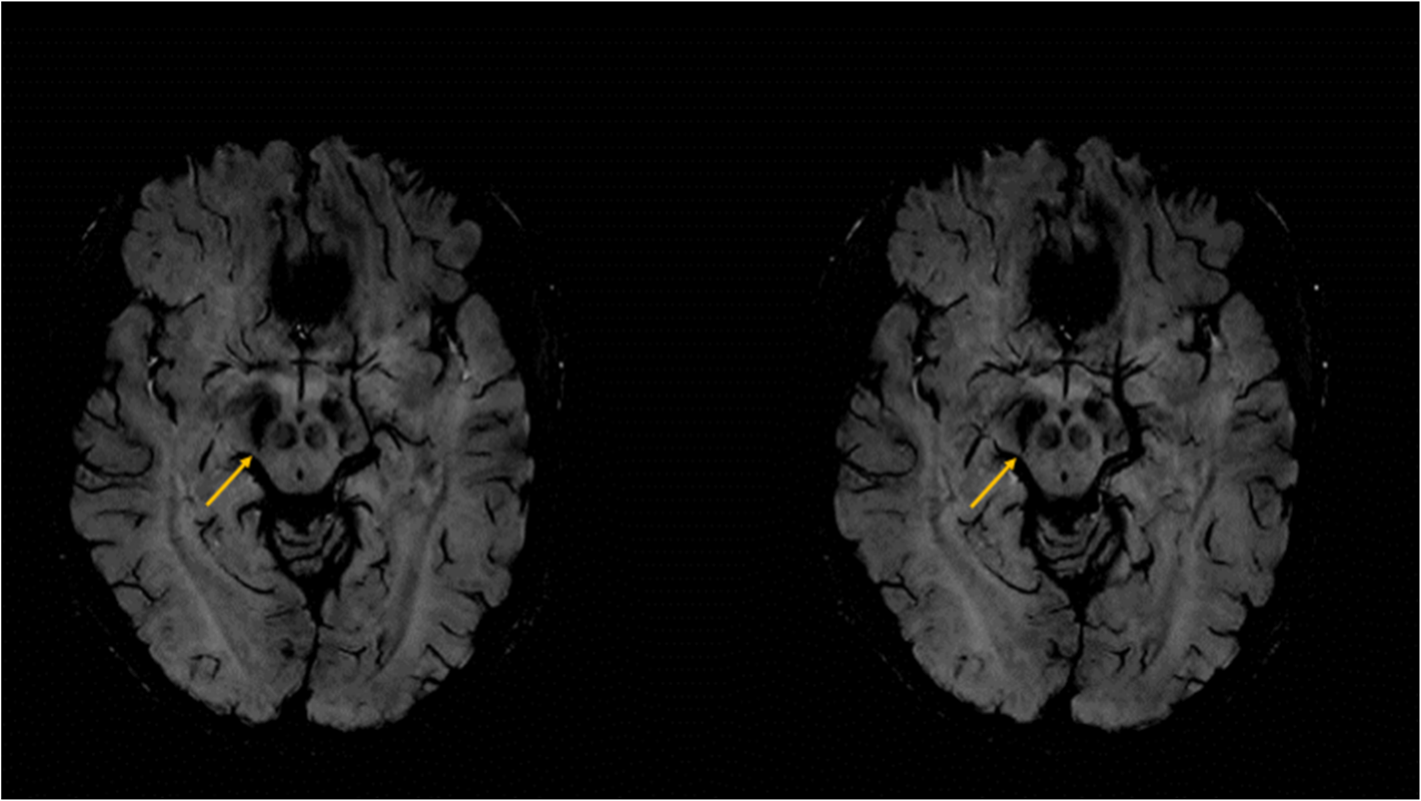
Brain MRI of the patient. On the axial susceptibility weighted images in the plane of the mesencephalon, the substantia nigra is identifiable both sides. The swallow tail sign is normally present in 3 T imaging at the area indicated by the arrows, but it is absent in the patient
